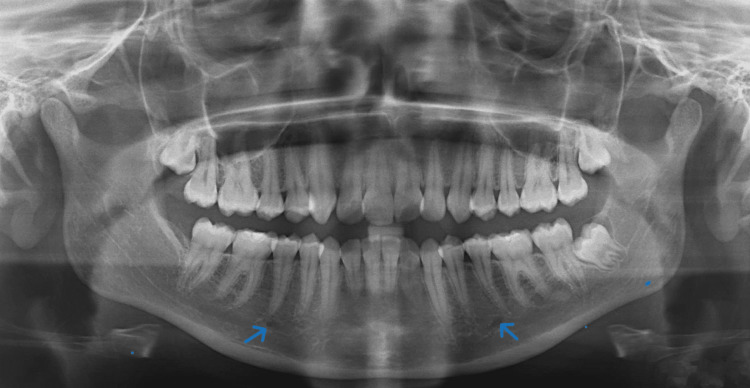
Panoramic radiograph showing MF on the fourth position and shape (blue arrows) MF: mental foramen
